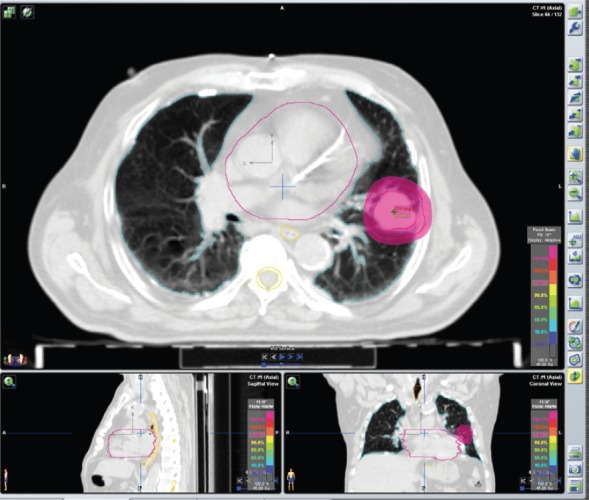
Plan of SBRT Left lung cancer, stage: cT2 cN0 cM0. SBRT treatment plan: Two dynamic arcs, Energy: 6MV XRays. Total dose: 45 Gy in three fractions (15 Gy/fraction) every other day.
